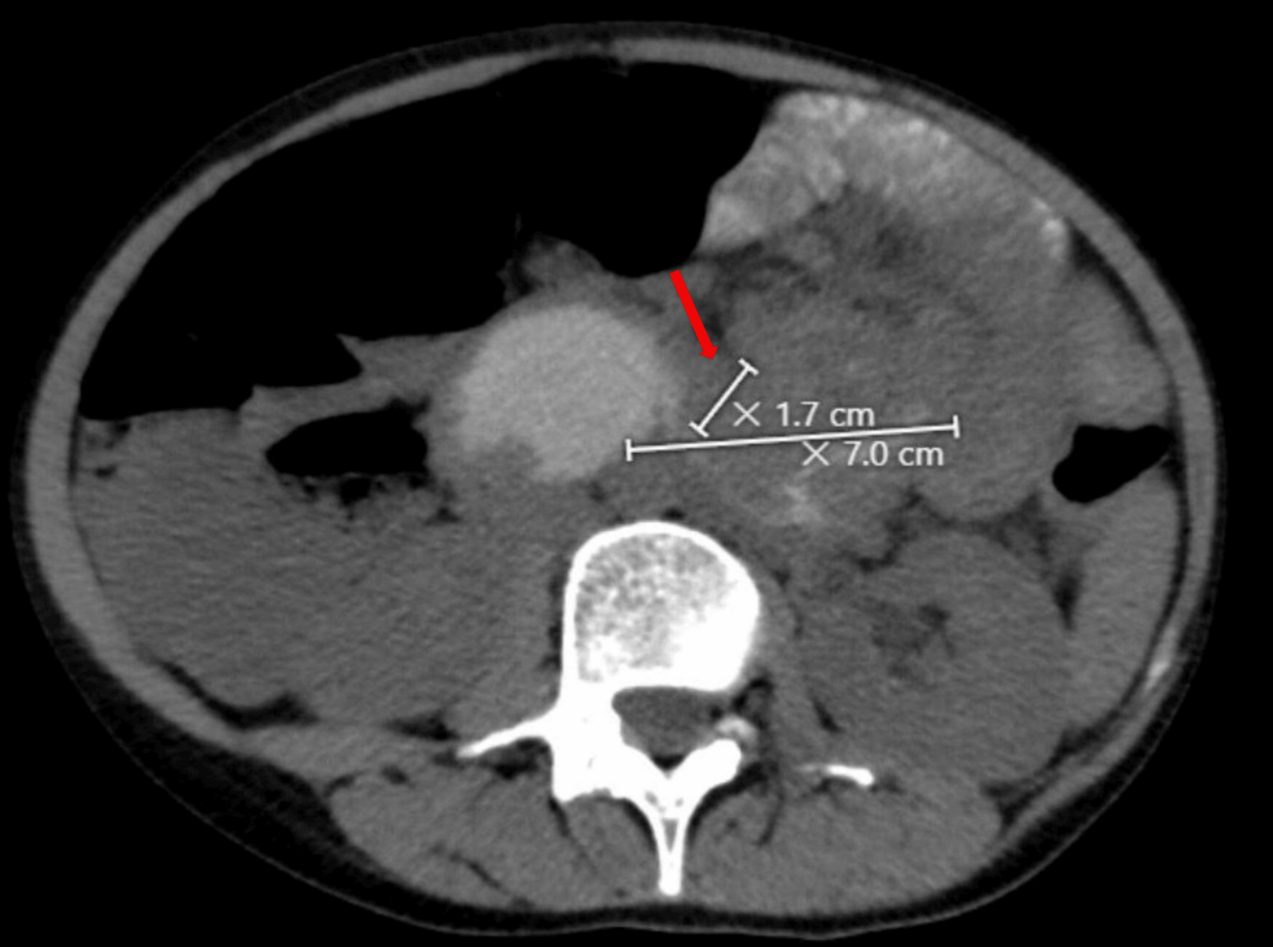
Abdominal CT scan with oral contrast showing irregular circumferential thickening involving a long segment of the proximal jejunal small bowel loops with maximum thickness measuring up to 1.7 cm.
